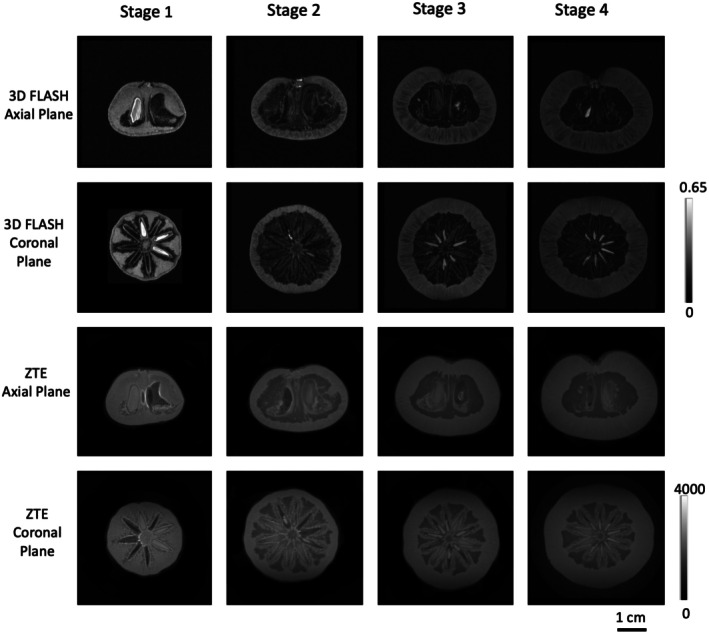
Axial and coronal plane of 3D FLASH and ZTE. MR image slices were shown for the middle slice of Burdekin plum. The gray scale maps indicate image intensities in arbitrary unit.
